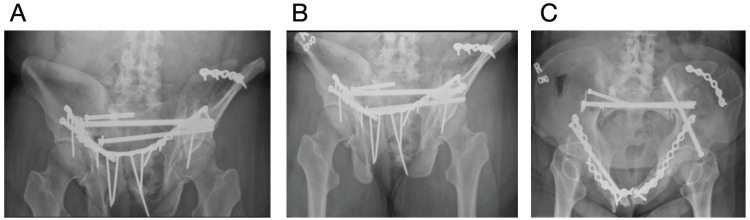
Three-month status post revision surgery radiographs Images A (AP pelvis), B (pelvic outlet), and C (pelvic inlet) show the anterior plate to be broken in two different places with anterior displacement. The images also show a broken left LC-2 screw at the level of the iliac wing.
